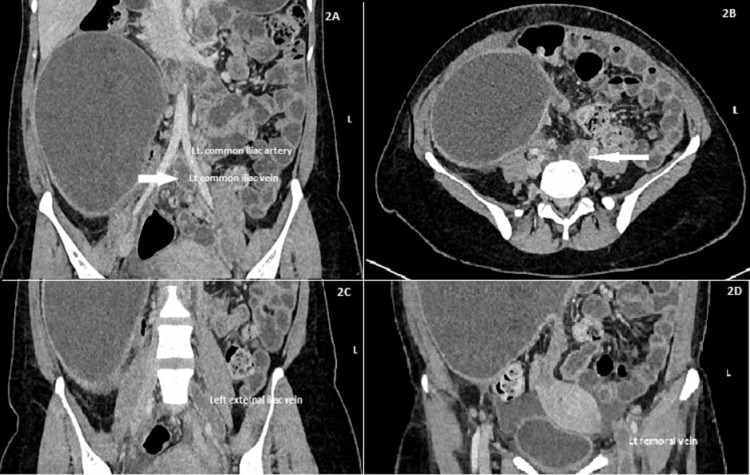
CT venography images CT venography (A) coronal section and (B) axial section showing left common iliac vein thrombosis (white arrow) and compression by left common iliac artery suggestive of May-Thurner syndrome; coronal sections showing the extent of thrombosis to (C) the left external iliac vein and (D) the left femoral vein.
